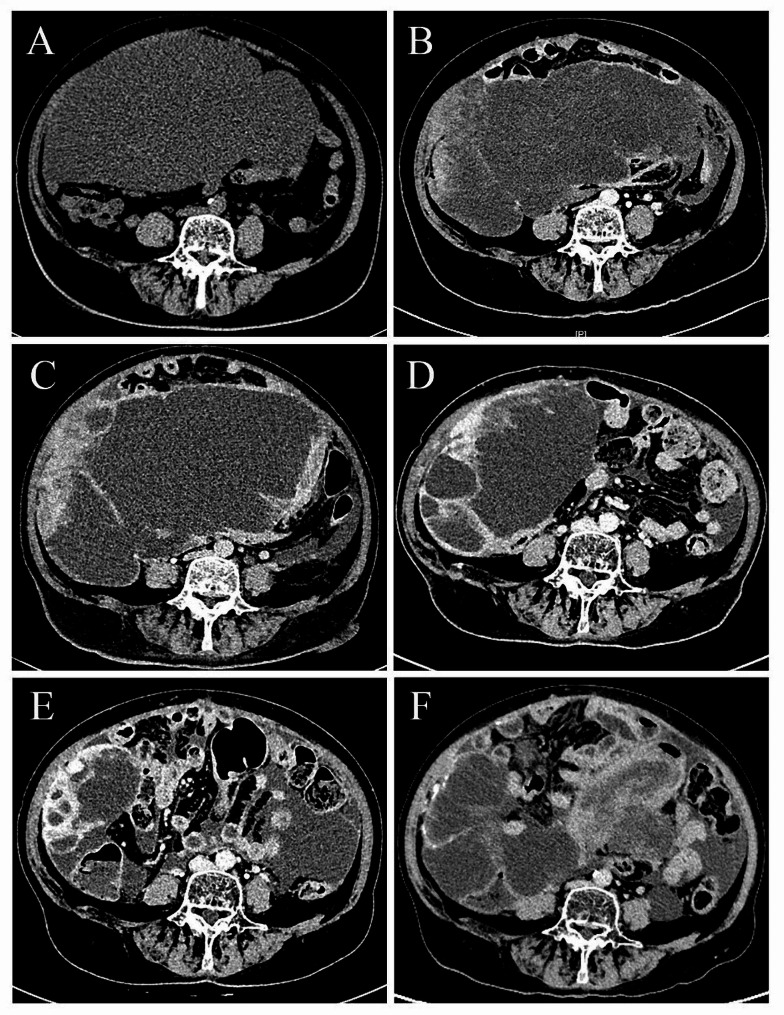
Radiologic features at different time points. A Preoperative CT scan (unenhanced CT) (2022-4). B Tumor recurrence after surgery (enhanced CT) (2022-6). C After 2 cycles of chemotherapy, no significant relief was revealed using enhanced CT (2022-8). D After 6 months of pamiparib treatment, an enhanced CT scan indicated PR (2023-2). E A 10-month PFS was achieved using pamiparib (enhanced CT) (2023-6). F The latest enhanced CT review (2023-9)
